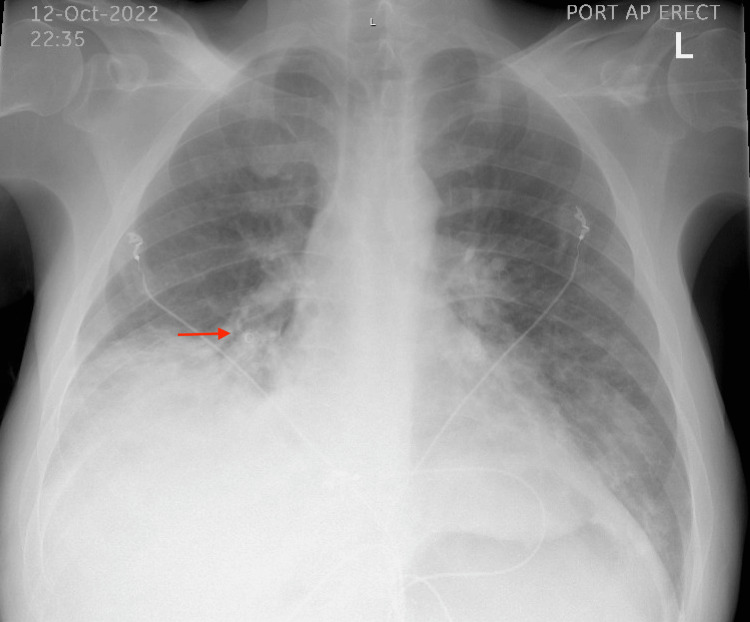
Anteroposterior chest X-ray showing right-sided multifocal pneumonia
